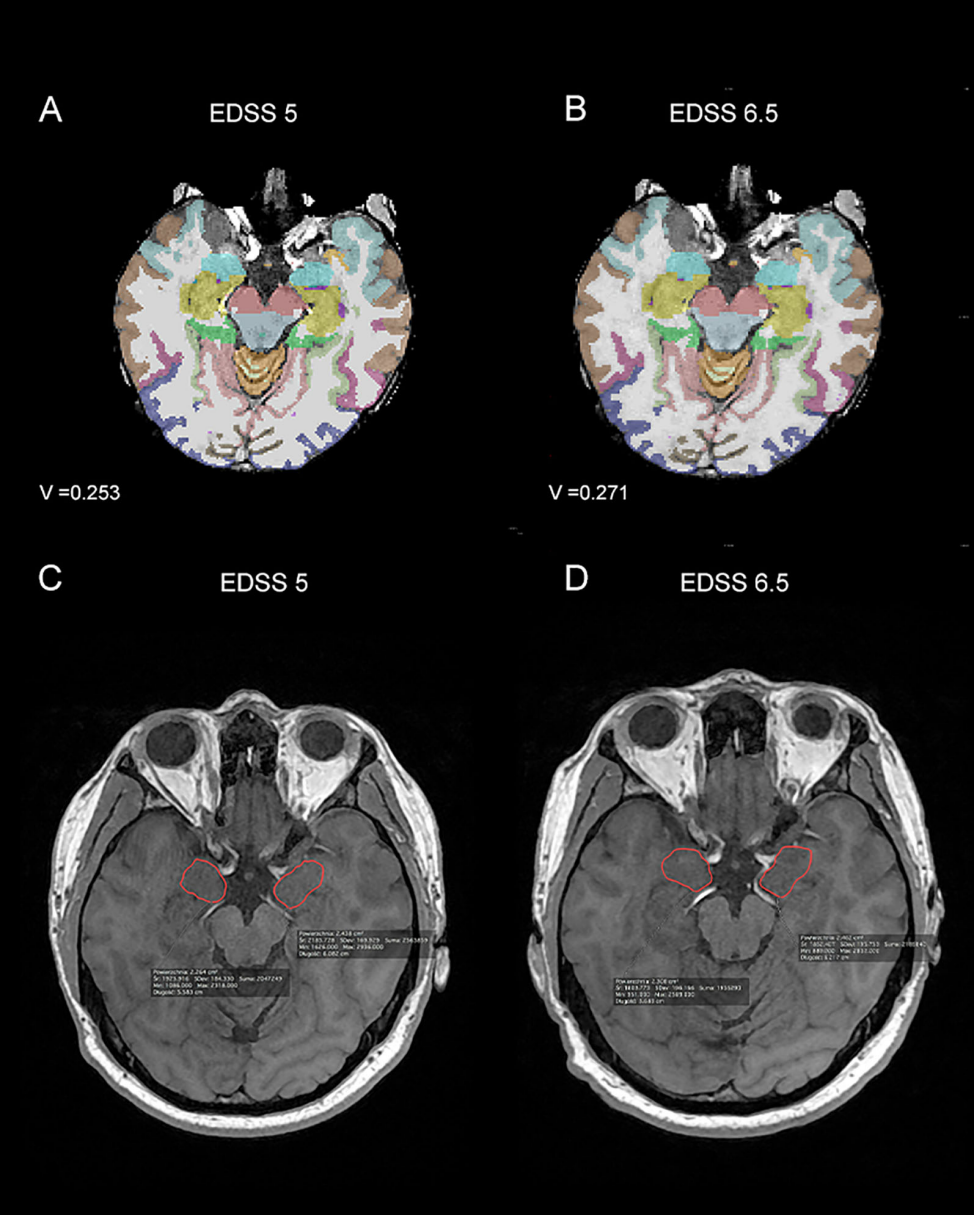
Magnetic resonance imagining (MRI) scans from patient with Expanded Disability Status Scale (EDSS) progression - at time point T0 (A, C) the EDSS was 5, while at time point T1 (B, D) it was 6.5. In parts (A, B) images from Freesurfer software at time points T0 - baseline (A) and T1 – after one year of treatment (B) with the amygdala volume (V) marked were shown. In parts (C, D) 3D T1-weighted MRI images from the Alteris system at time points T0- baseline (C) and T1 – after one year of treatment (D), with the dimensions of the amygdala marked manually by the radiologist, were shown. V, volume of amygdala.
